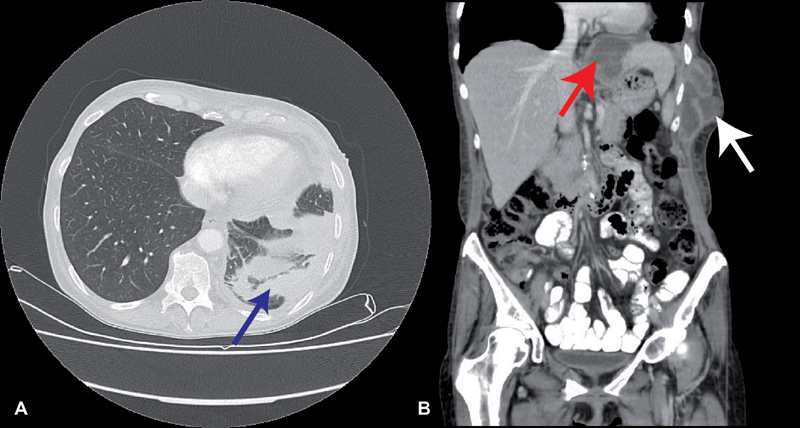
CT-scan of 61-year-old female showed a pleural empyema (
